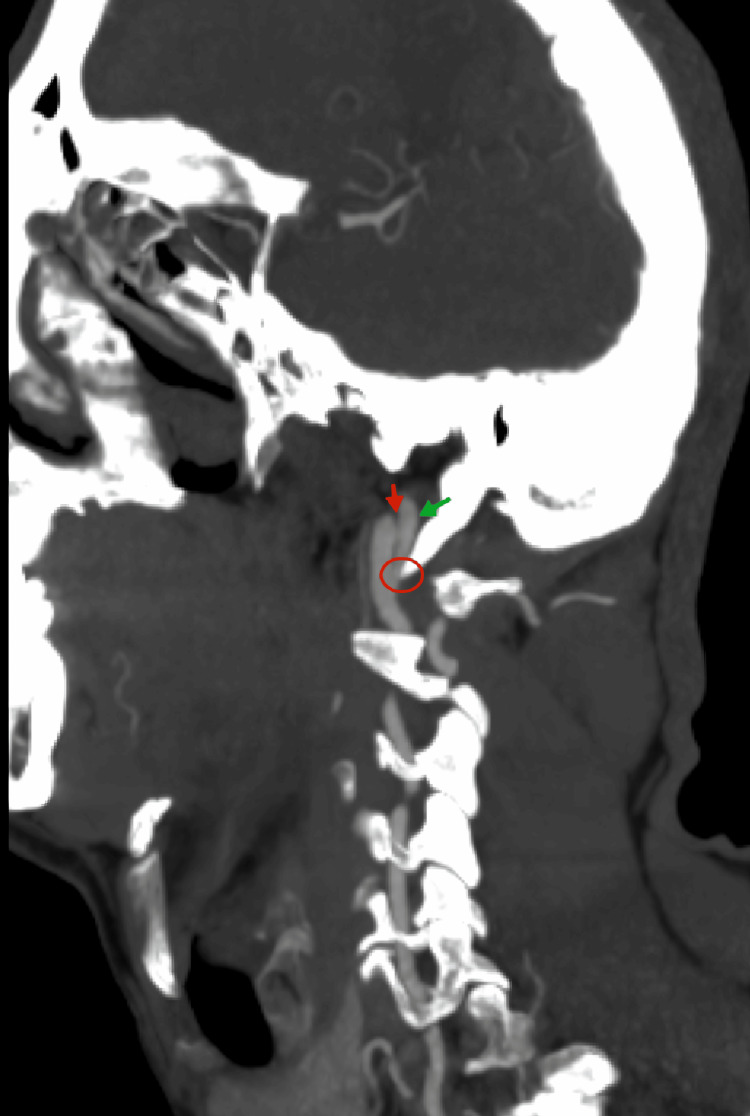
The computed tomography angiography (CTA) sagittal oblique view demonstrated an elongated styloid process (ESP) impinging on the right internal carotid artery (ICA) (indicated by the red circle), causing an intimal flap tear (red arrow) and a pseudoaneurysm (green arrow).
